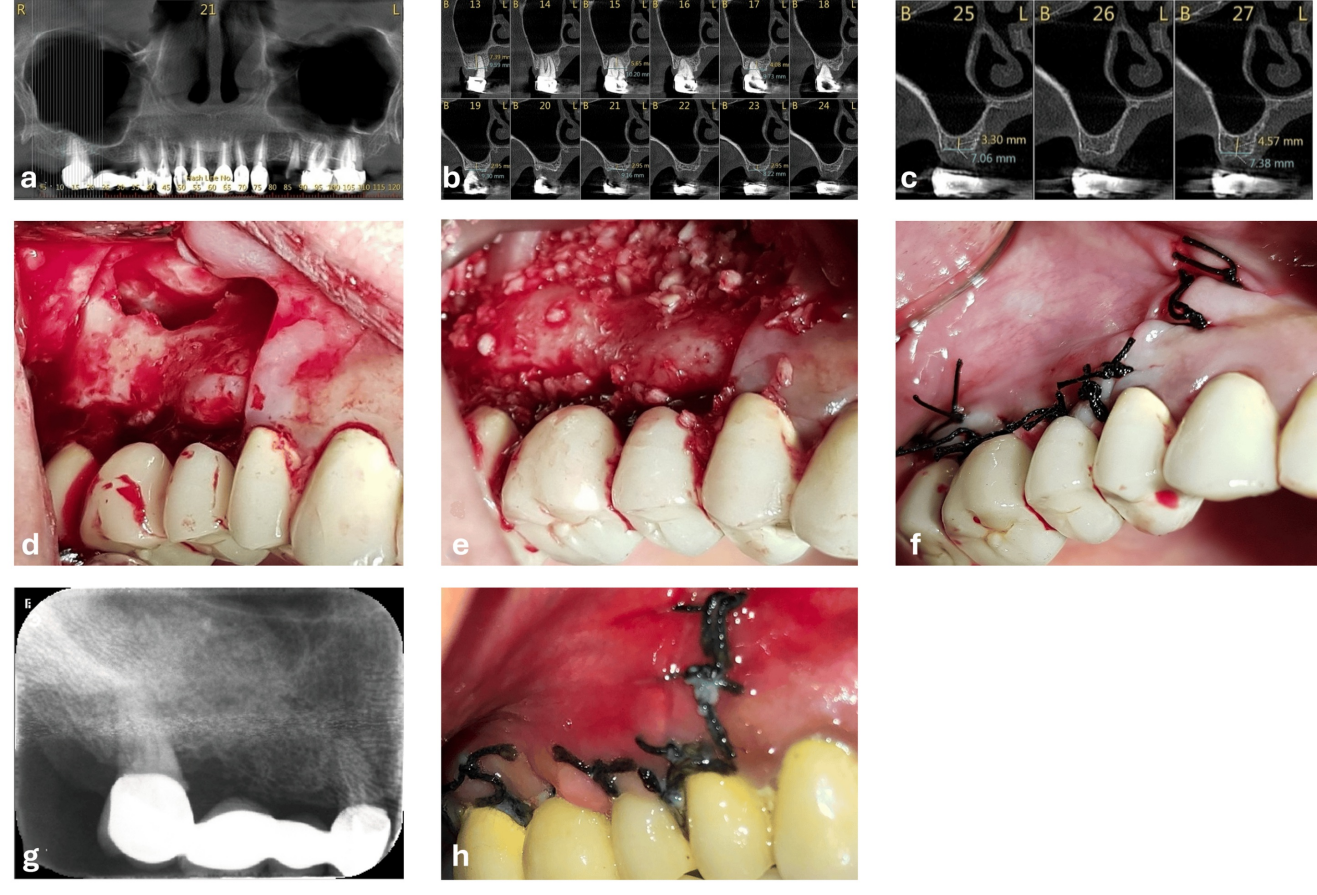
Clinical and radiographic presentation of case 1. (a-c) Preoperative cone-beam computed tomography images showing vertical bone deficiency at the right maxillary sinus region. The first premolar and second molar served as abutments for a fixed prosthesis, while the second premolar and first molar were missing. (d) Access to the maxillary sinus following envelope flap elevation. (e) Sinus augmentation was performed through the lateral window approach. (f) Primary flap closure achieved without tension. (g) Postoperative radiograph showing the augmented sinus; the existing fixed prosthesis was retained temporarily during the healing phase. (h) Clinical view at 10-day follow-up demonstrating intact mucosa and absence of wound dehiscence.
